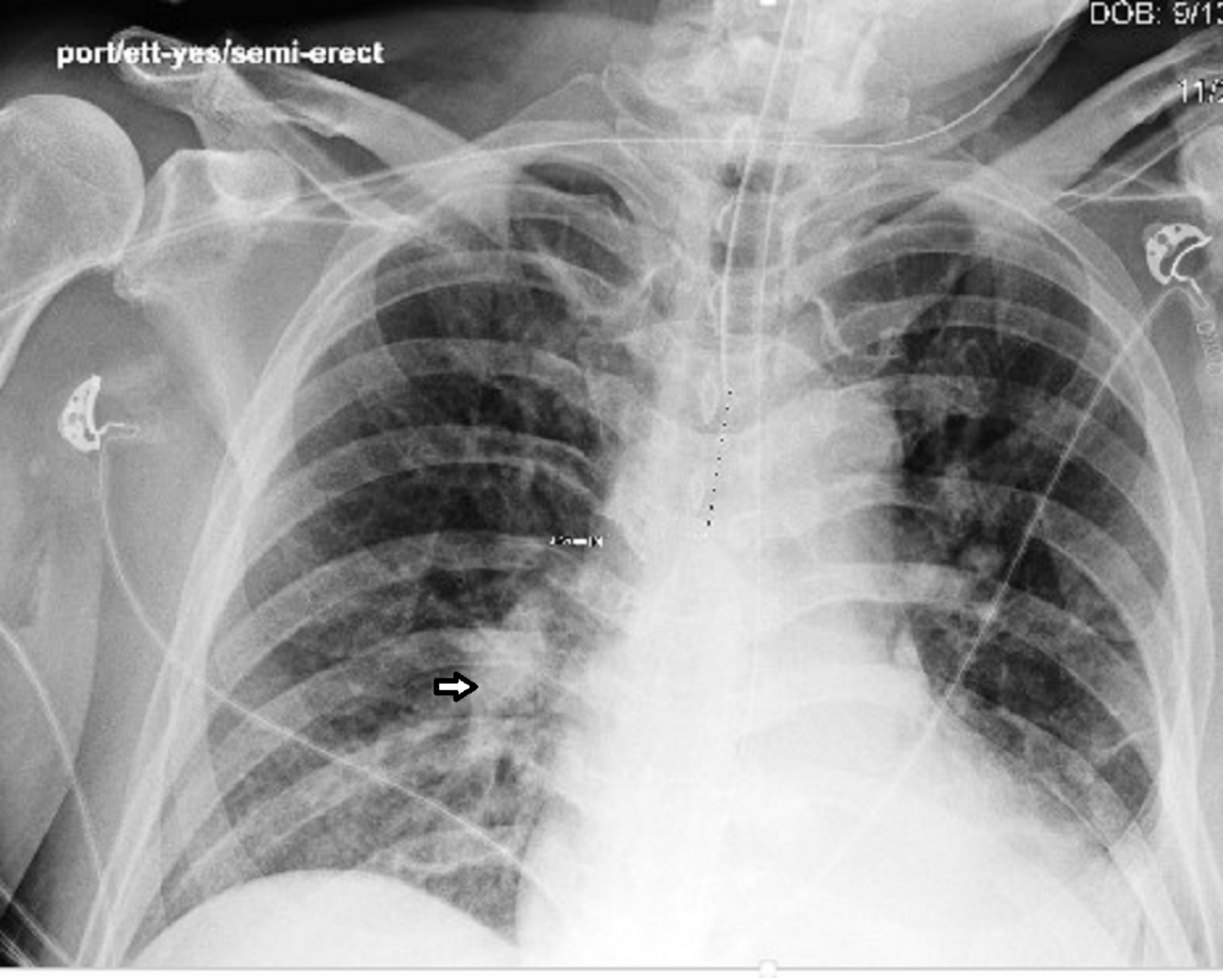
Chest x-ray showing the presence of a left lower lobe infiltrate
